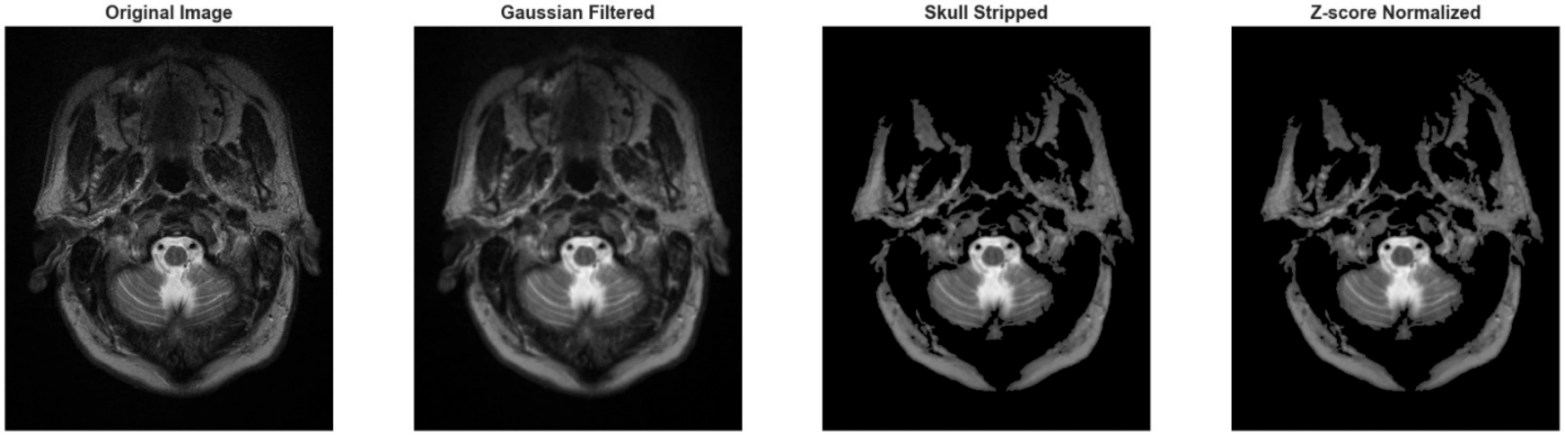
Preprocessed images of MRI.
